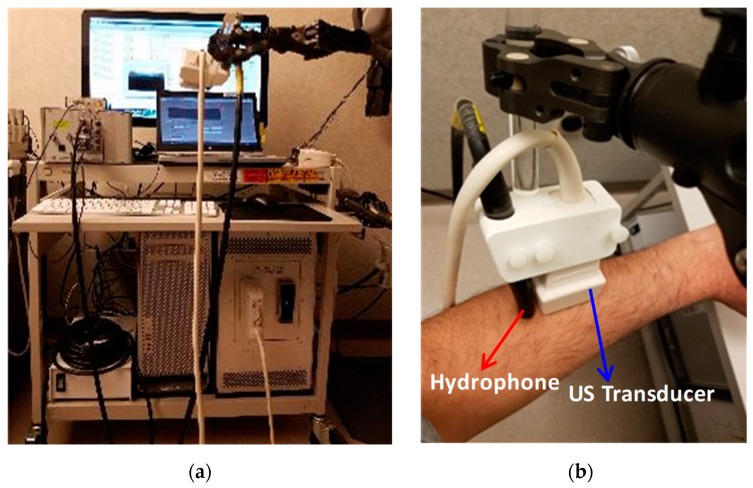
Experimental setup of URF bone measurement including: (a) Verasonics V-1 machine and probe-hydrophone assembly; (b) ultrasound transducer and hydrophone assembly placement on skin. Red arrow shows the hydrophone and blue arrow shows the ultrasound transducer.
